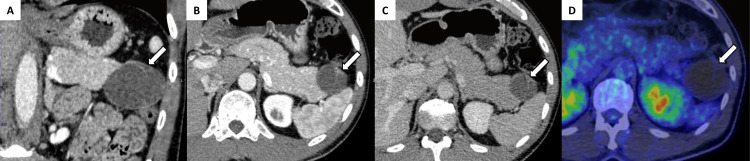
Computed tomography and 18F-FDG PET/CT imaging findings (A) Twelve months later, a coronal image of the late arterial phase reveals that the cyst had grown to 50 mm in diameter (arrow). The solid component continues to show enhancement similar to that of the spleen across all phases (arrows in B and C). B shows the late arterial phase, and C shows the portal phase. (D) 18F-FDG PET/CT showed no abnormal FDG uptake (arrows). 18F-FDG PET/CT: Fluorine-18 fluorodeoxyglucose positron emission tomography/computed tomography.
